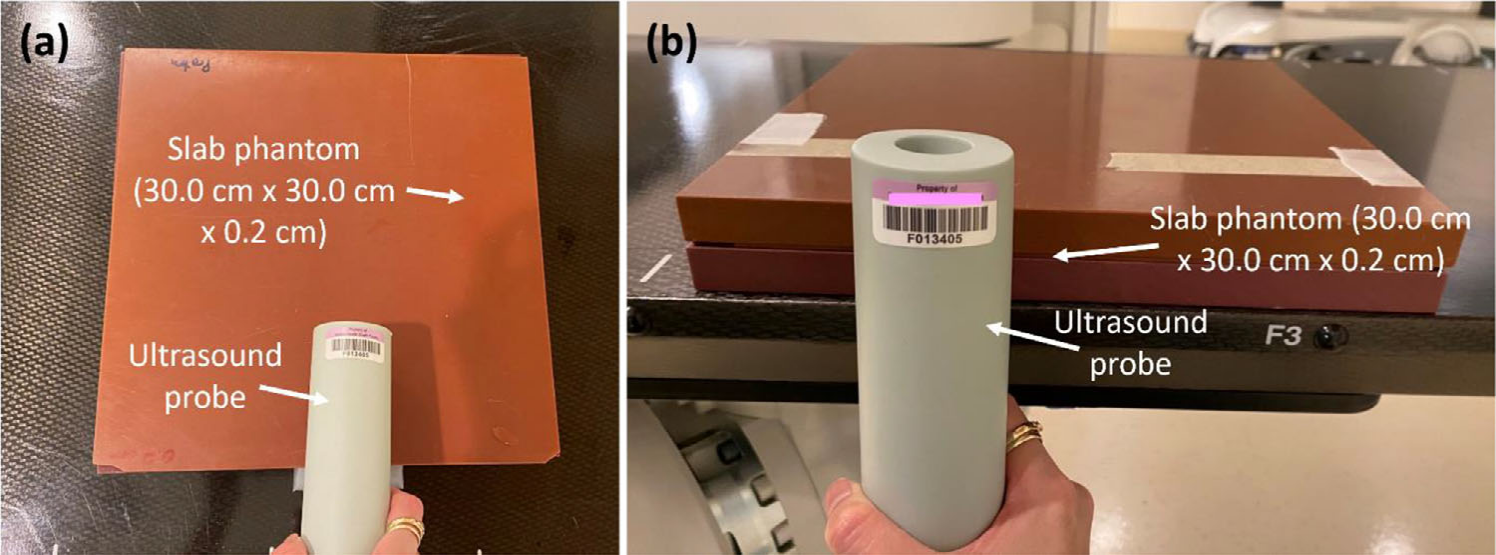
Setups for (a) depth and (b) width measurements on ultrasound images acquired in an SRT‐100 Vision unit.
